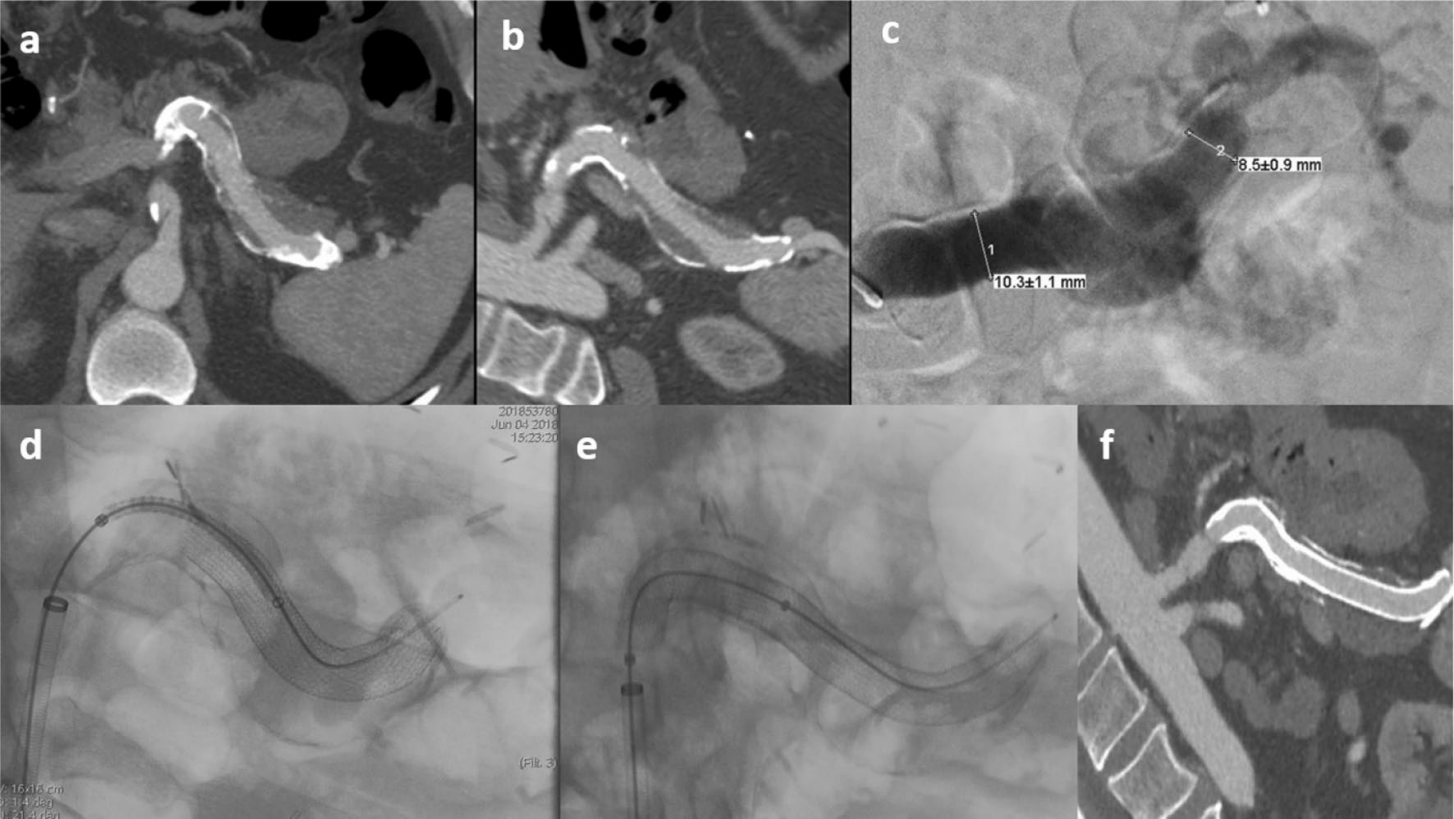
(a) CT scan in arterial phase demonstrated a 95 × 36 mm GAA of the splenic artery. (b) Curved reconstruction shows the complete extension of the aneurysm and the thrombosed part of the sack. (c) Angiogram confirming the aneurismal dilatation and allowing treatment planning. (d,e) Endovascular treatment was performed by positioning three overlapping covered stents (Viabahn; Gore, Falstaff, AZ) measuring in order from distal to proximal: 10 × 100 mm, 10 × 50 mm and 11 × 50 mm f) Angio-CT with curved reconstruction shows patency of the splenic artery with aneurysm complete exclusion.
